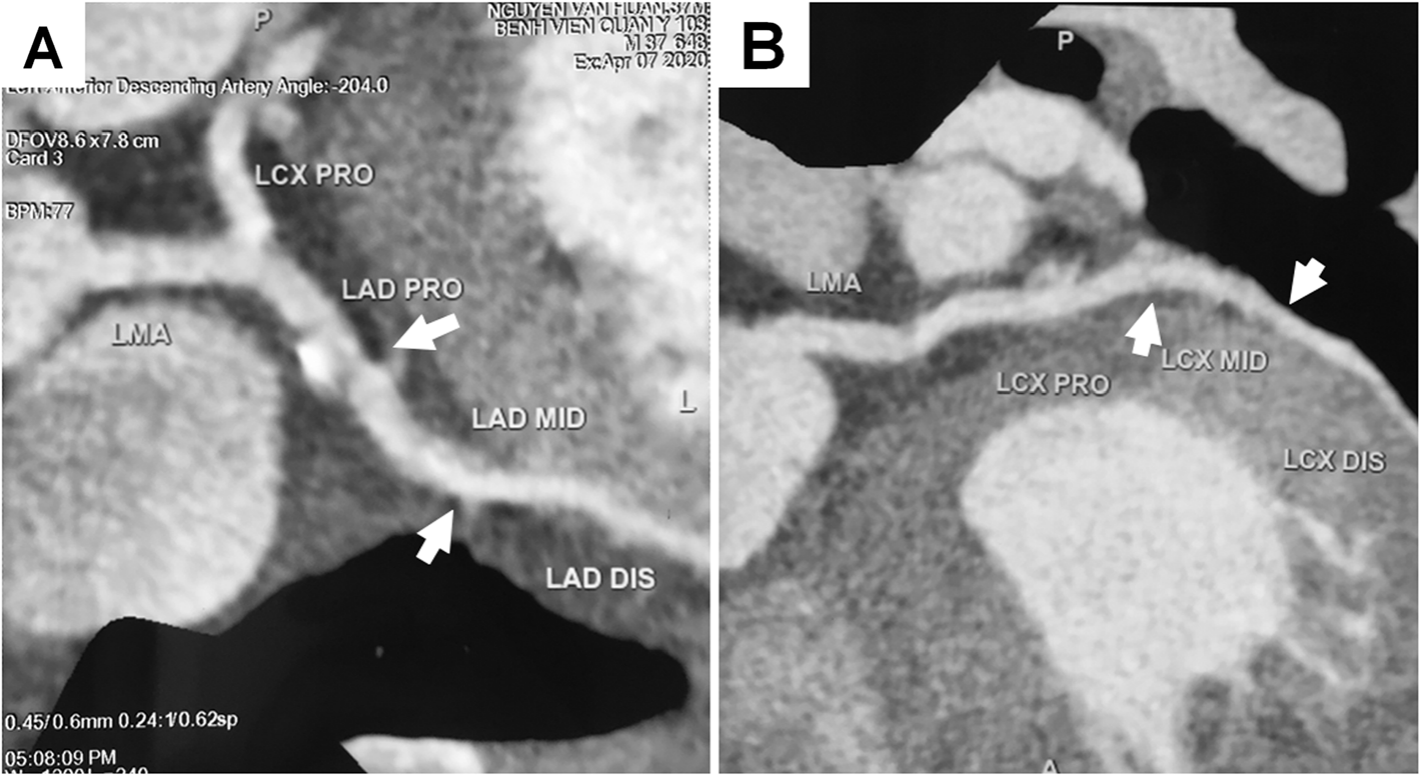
Postoperative CT coronary angiogram. Mild stenosis was noted at the middle left anterior descending coronary artery (LAD) (50%) and middle left circumflex coronary artery (LCx) (50%). LMA left main artery, PRO proximal, MID mild, DIS distal
